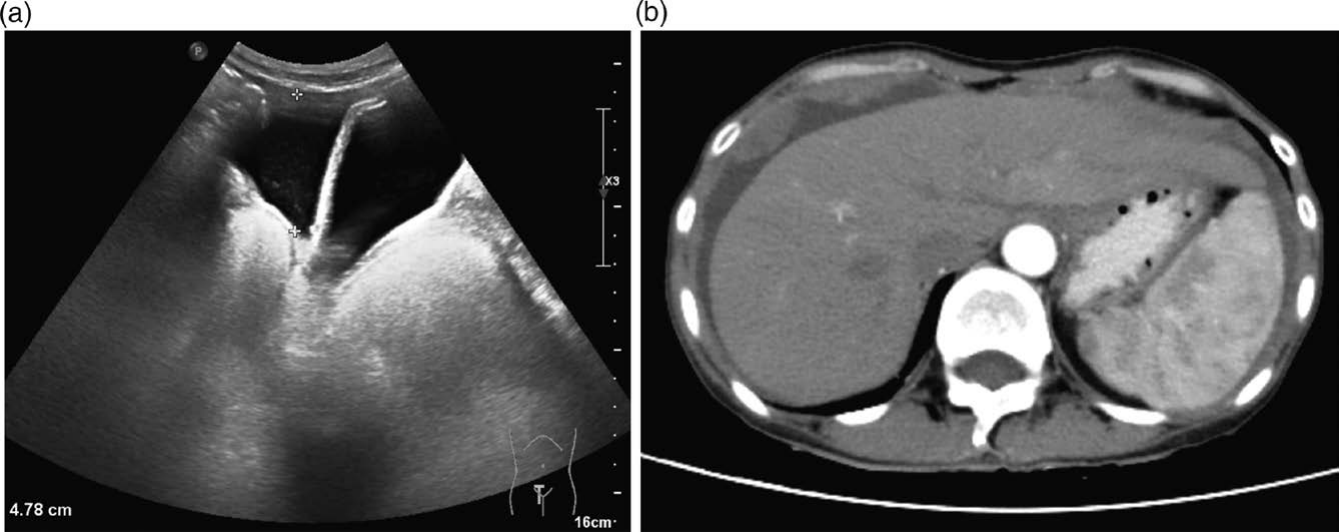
Imaging examinations after targeted therapy in the case. (a) Abdominal ultrasound images. (b) Abdominal CT results. CT, computed tomographic.
